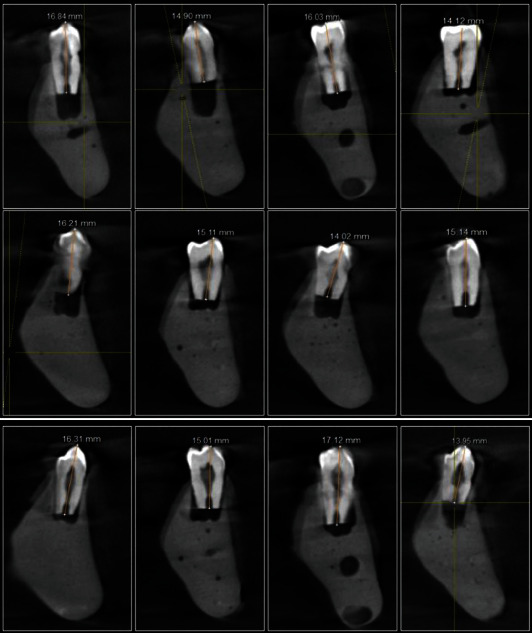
WL measurement of mandibular premolars using CBCT imaging (CWL).
